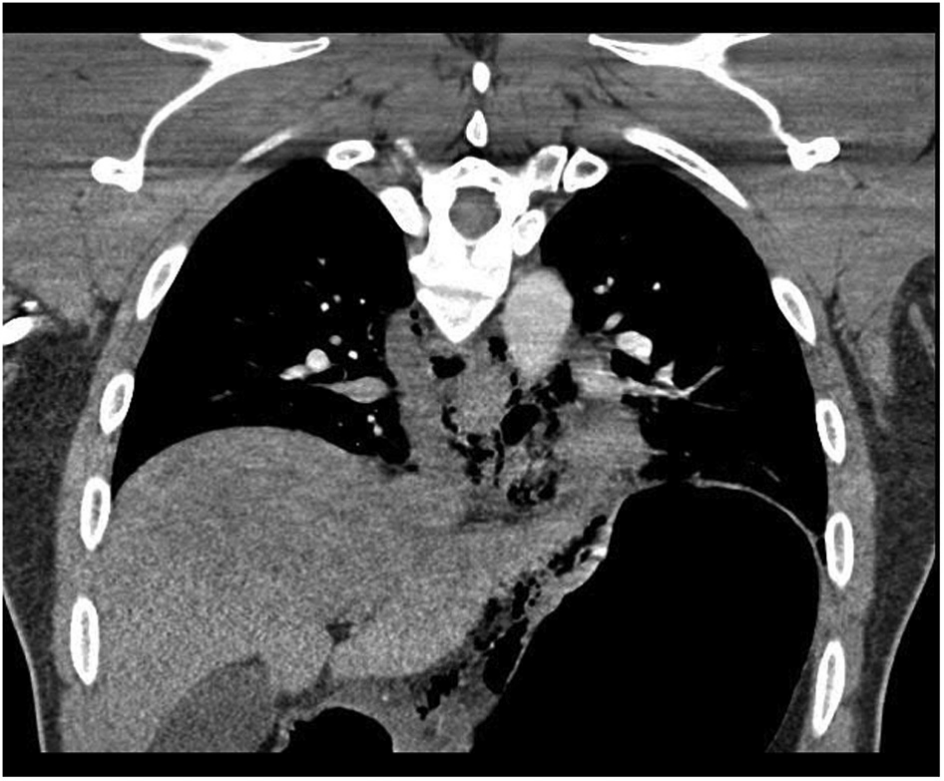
Chest CT scan showing a complex heterogenous air and fluid-filled mediastinal collection.
